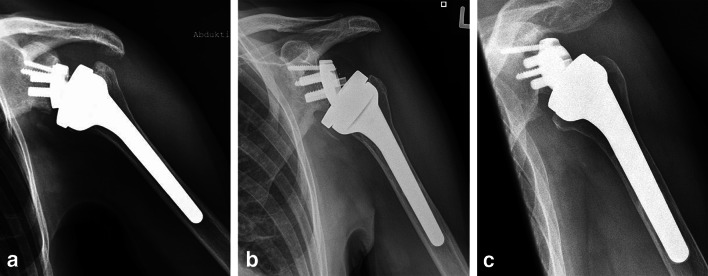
X-ray of the evaluated prosthesis: a initial notching (grade 1) on the inferior rim of the scapular neck. b Grade 1 notching. c Grade 2 notching. Note that the shape of the notch matches the shape of the humeral inlay, and the bone defect is located away from the metaglene without any baseplate contact
